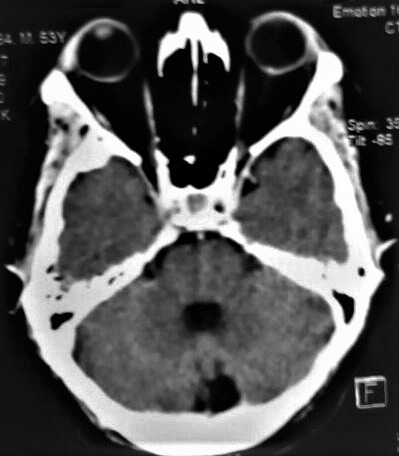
Computed tomography scan of the brain and orbit showing hyperdense lesions in both optic nerve heads larger on the left side
